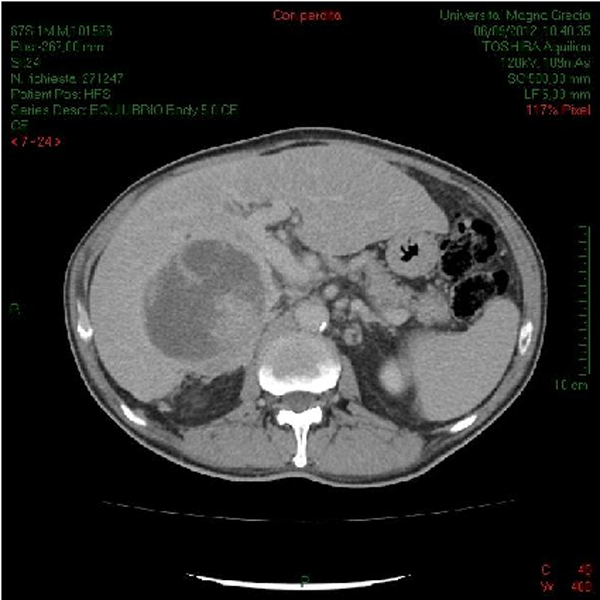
Abdominal contrast enhanced CT scan NOTE: CT scan showed mixed hypo-isodense lesion with regular borders and enlarged lymphonodes of approximately 1.5 cm at the hepatic hilum.
